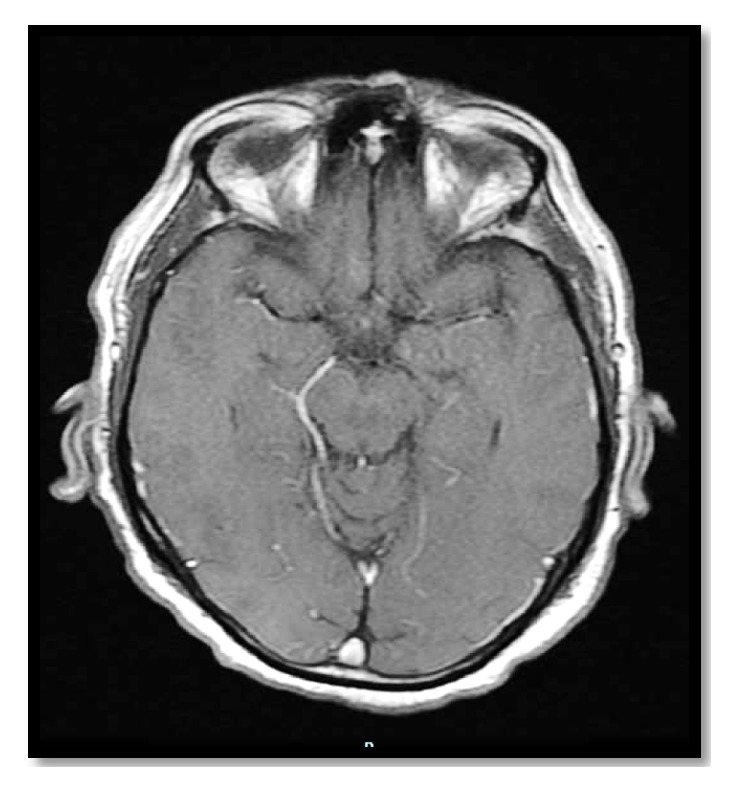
Periictal brain MRI with contrast showing no corresponding area of enhancement. Abbreviation: MRI, magnetic resonance imaging.
